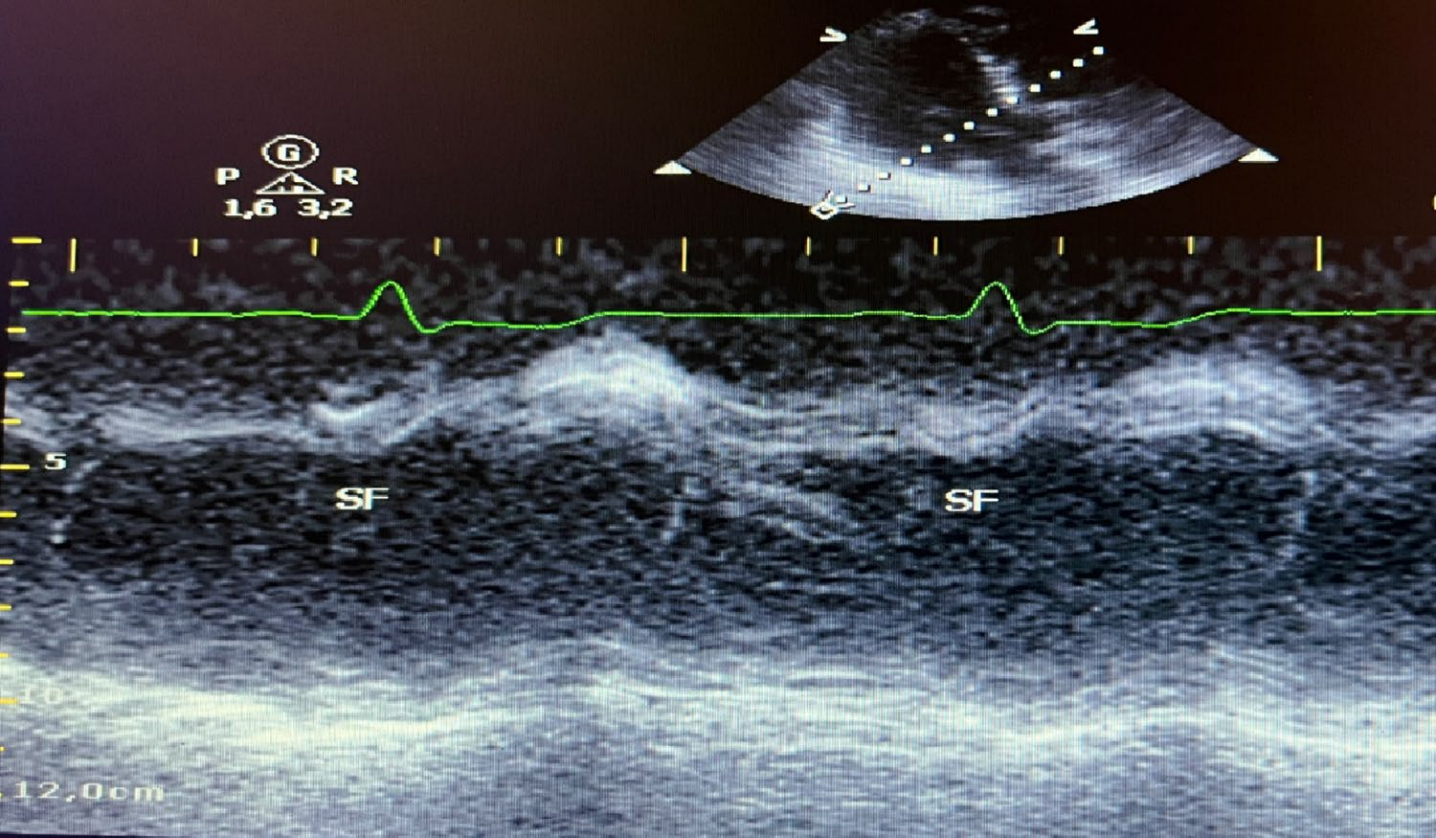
Example of Septal flash (SF) simply identified on 2D echocardiography using M-Mode, SF represented as a short inward early systolic motion of the septum due to the early septal excitation and contraction
